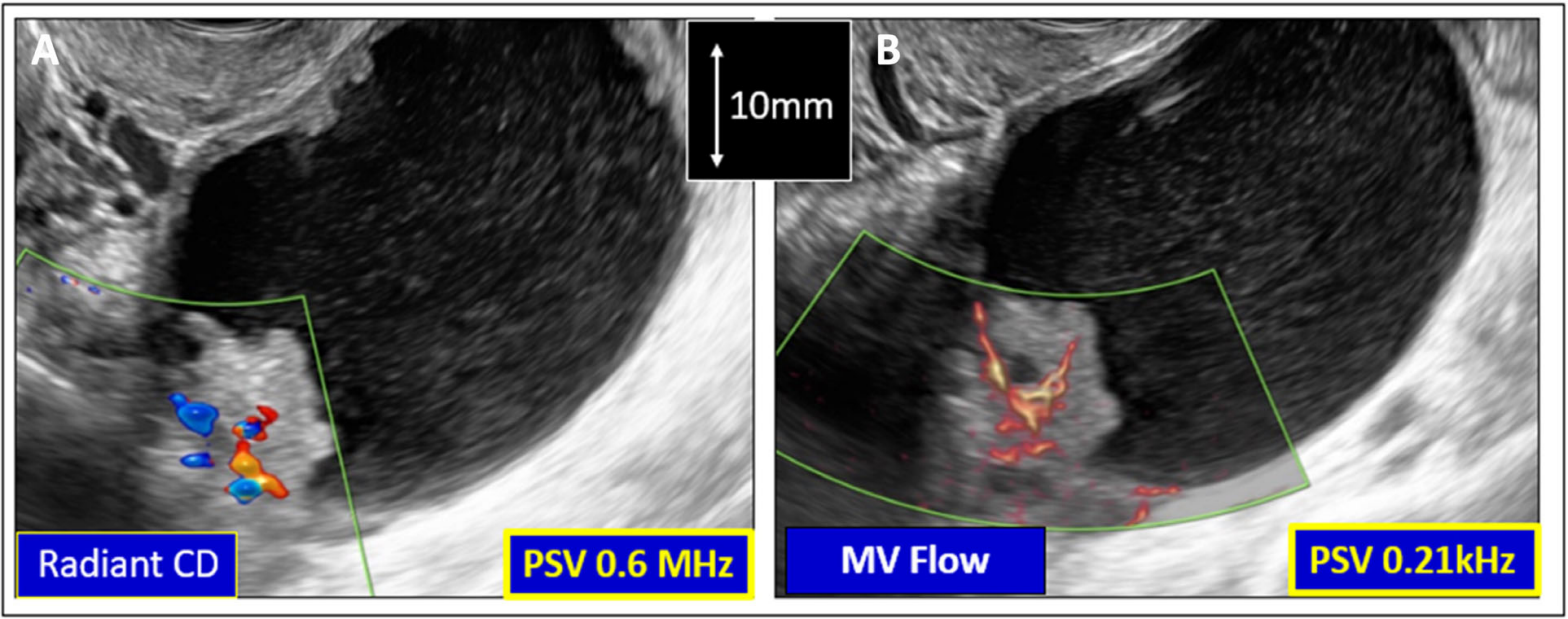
Ultrasound imaging of serous borderline ovarian tumor using radiant color Doppler US (CDUS) with a PRF of 0.6 kHz and microvascular imaging (MVI) using a PRF of 0.21 kHz. Note the difference in the details of the vascular patterns.
